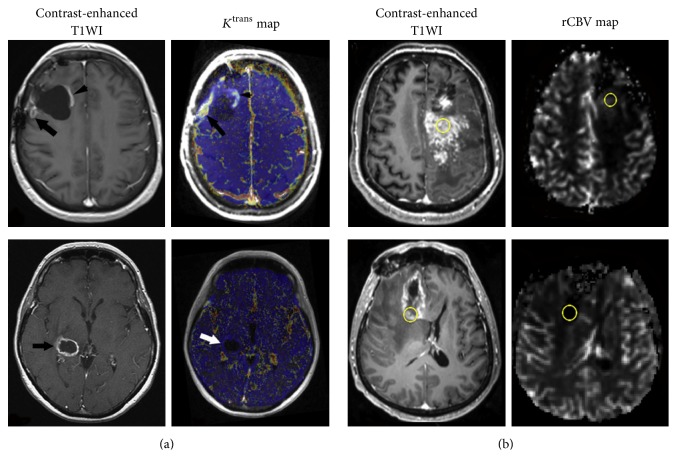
Discrimination of RN from recurrent GBM using DCE-MRI (a) and DSC-MRI (b). Contrast-enhanced T1WI demonstrates similar contrast enhancement in recurrent glioblastoma* (top row)* and RN* (bottom row)*. Corresponding rCBV and *K*^trans^ maps show significant difference between these two entities, with higher *K*^trans^ and rCBV for recurrent tumor* (top row)* but low for RN* (bottom row)*. Reproduce with permission from Bisdas et al. [184] and Masch et al. [186].
